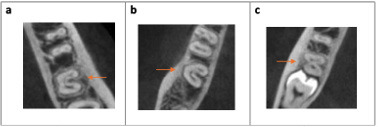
CBCT images of C-shaped molars. The arrows indicate the buccal surface. (a) Buccal longitudinal groove, (b) Lingual longitudinal groove, and (c) Longitudinal grooves on both buccal and lingual walls.
